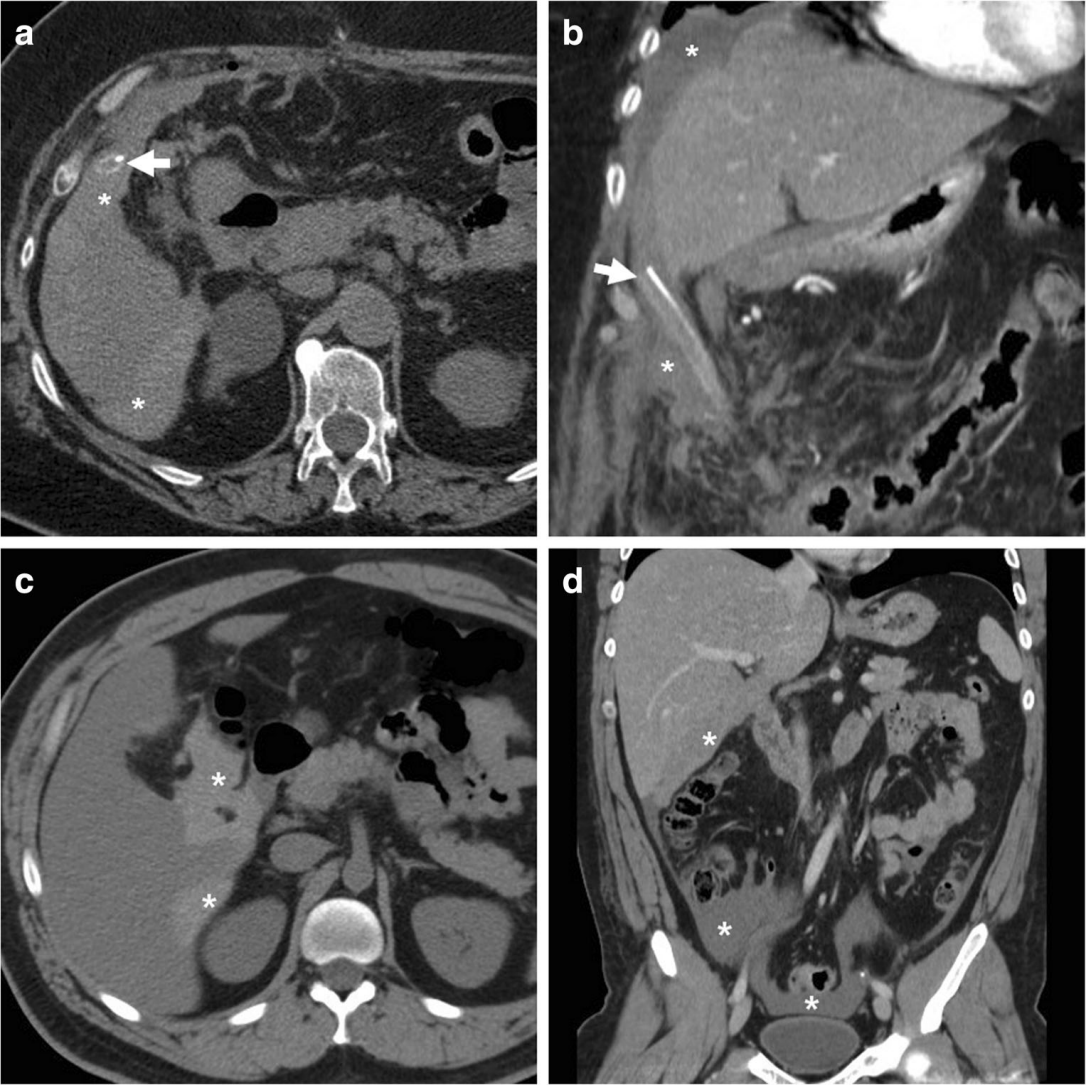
The most common appearance of post-cholecystectomy bleeding. a, b Forty-eight hours after open cholecystectomy converted from laparoscopic cholecystectomy, CT showed perihepatic blood (*) isoattenuating with the liver on precontrast scans (a), oozing externally from drainage (*thick arrows*), without appreciable contrast extravasation on both arterial (b) and venous (not shown) enhanced phases. Reintervention was required to achieve haemostasis at the gallbladder fossa and omentum. c, d On the 3rd postoperative day after laparoscopic cholecystectomy, precontrast (c) and contrast-enhanced (d) CT images showed hyperattenuating fresh blood (*) extending from the surgical bed below the right liver lobe, in the right parietocolic and peritoneal cul-de-sac. The patient was treated by positioning of percutaneous drainage
